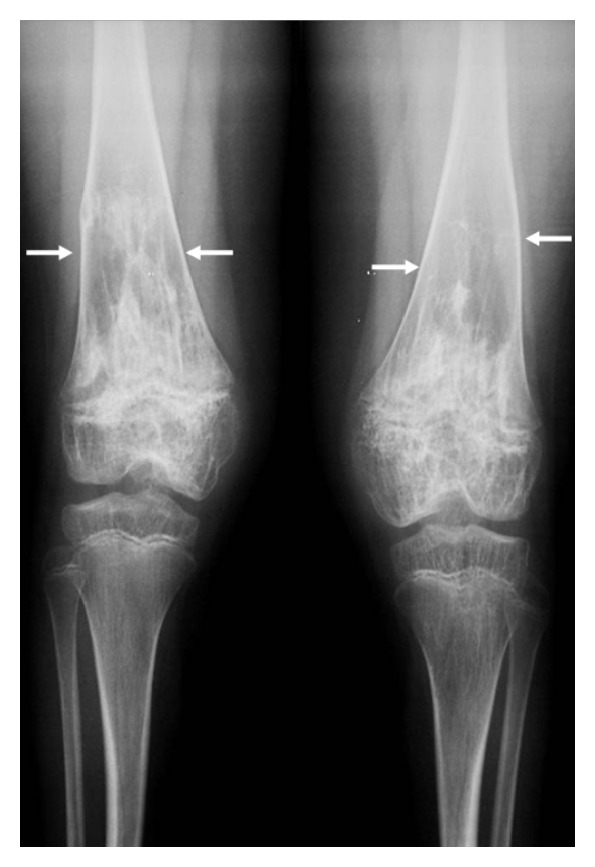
Anteroposterior radiograph performed in a 12-year-old boy with chronic infantile neurological cutaneous and articular (CINCA) syndrome, showing a calcified mass-like region in the physis of the distal femur (see white arrows). Osteoarthropathy of CINCA patients is a unique feature of this condition, caused by abnormal endochondral bone growth, mainly affecting large joints and long bones, beginning in the first infancy and causing striking changes until skeletal maturity.
